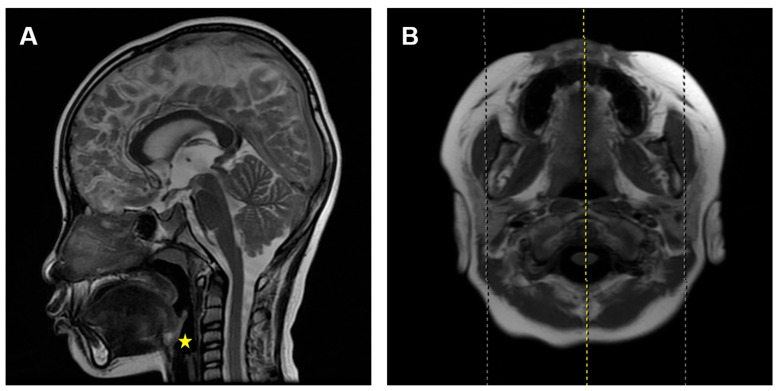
Mid-sagittal and cross-sectional head MRI images in T2W1 signal. (A) Mid-sagittal image. (B) Cross-sectional image. The yellow line represents the cross-sectional cutting position of the yellow star.
